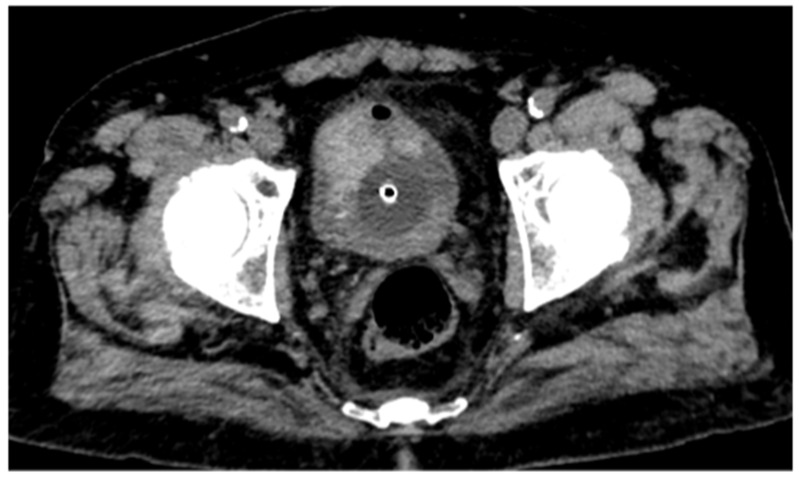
Conventional unenhanced CT scan shows a large hyperdense lesion on the anterior bladder wall and a mildly hyperdense area along the left posterolateral wall. Further evaluation with intravenous contrast medium administration is required to better characterize these findings.
